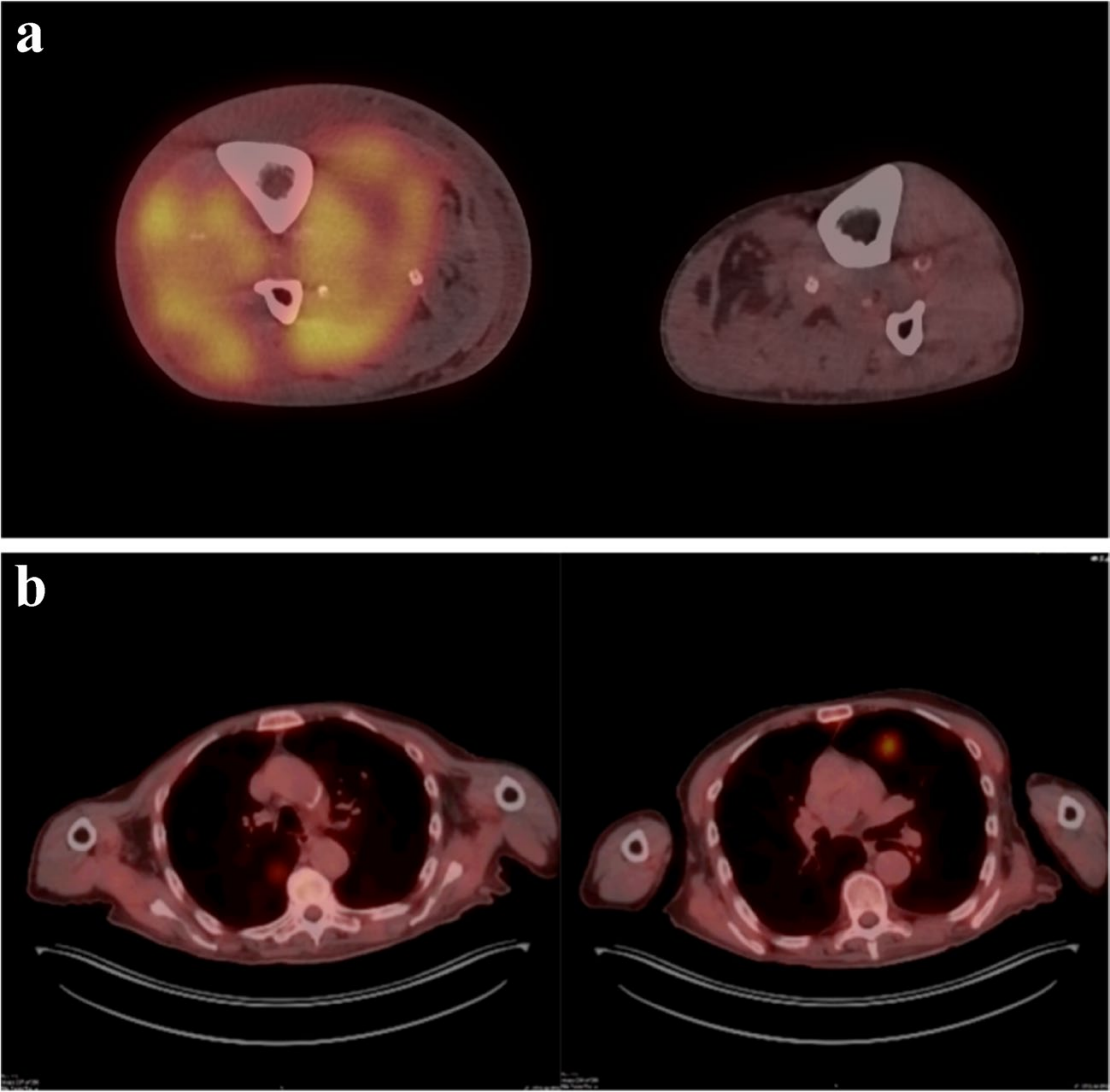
a (top) Fused FDG PET\CT images of the lower legs in the axial plane, revealing a noticeable difference in size between. There is significant swelling in the right lower leg, accompanied by increased FDG uptake in both the affected muscles and subcutaneous tissues. b (bottom) Fused FDG PET\CT images of the lung showing multiple lung lesions
